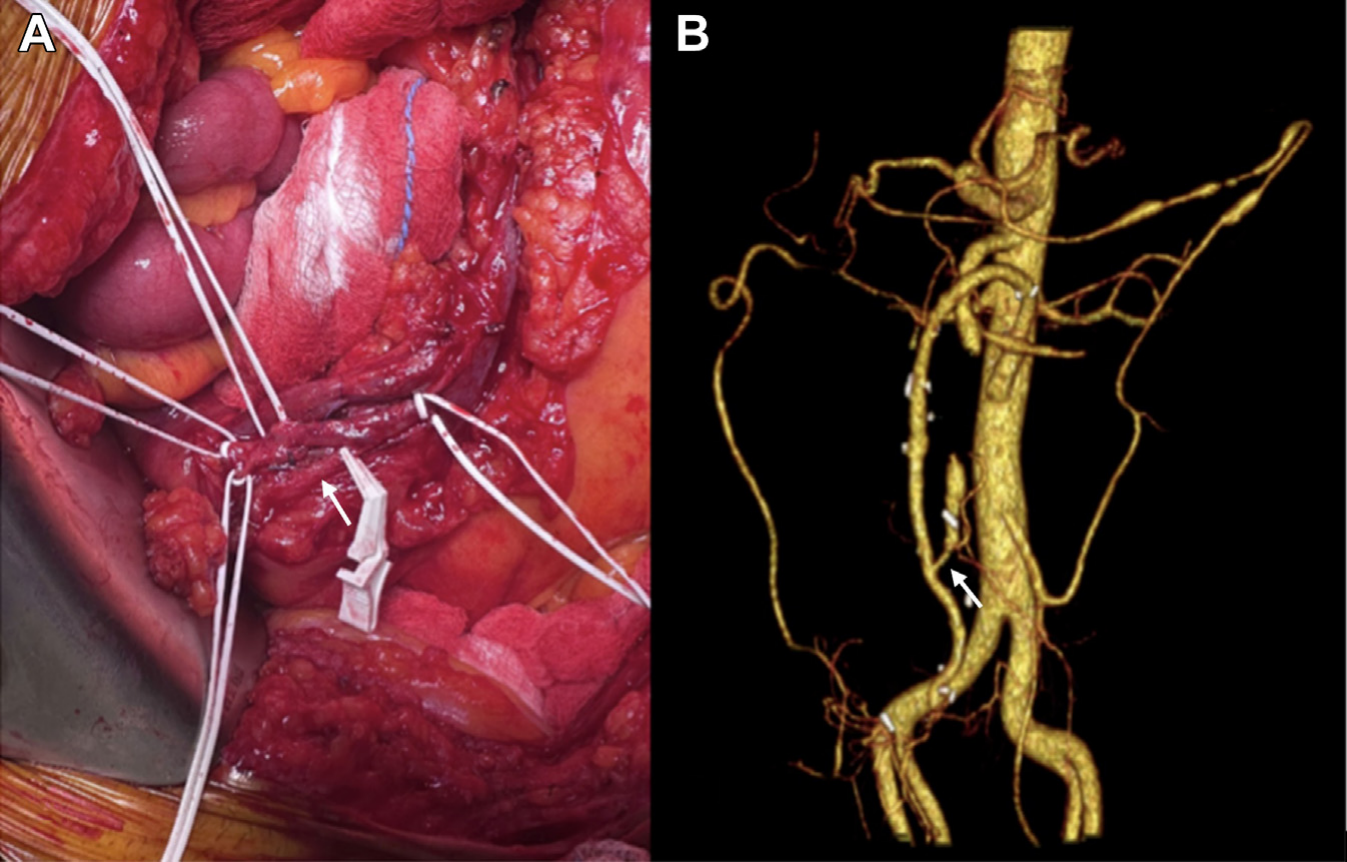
Case 1: Intraoperative and Postoperative Findings of Aortomesenteric Bypass with Ileocolic Branch Reimplantation (A) Intraoperative images showing the aortomesenteric bypass using the great saphenous vein and reimplantation of the first ileocolic branch (arrow). (B) Postoperative computed tomography angiography confirming the patency of the aortomesenteric bypass and the reimplanted branch (arrow).
